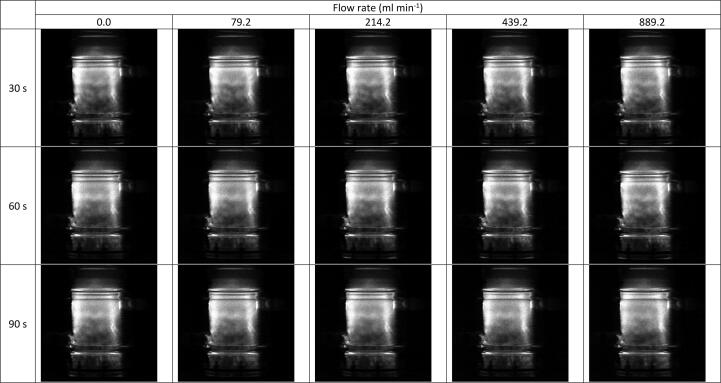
Sonoluminescence images for the sonolysis of 420 ml of Milli-Q water using 410 kHz ultrasound at 200 W L^−1^ applied power, at liquid flow rates of 0.0, 79.2, 214.2, 439.2, and 889.2 ml min^−1^, left to right respectively, and times of 30, 60, and 90 s, top to bottom respectively.
